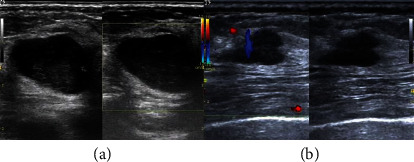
Breast tumor images acquired with two-dimensional gray scale ultrasound and color Doppler ultrasound: (a) malignant tumor image; (b) benign tumor image.
